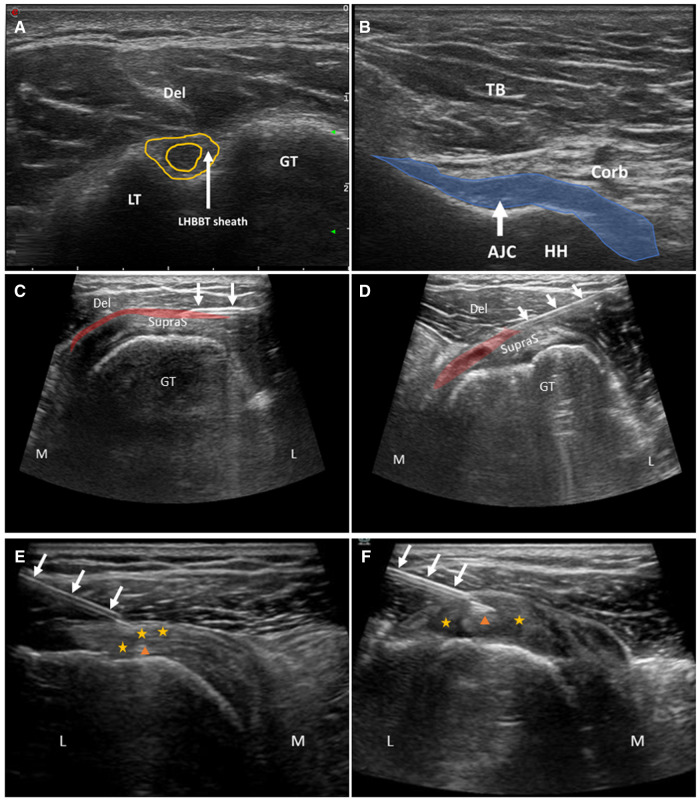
A,B are ultrasound images for diagnosis. C (before injection) and D (after injection) are US-guided injection into the subacromial Bursa. E (before injection) and F (after injection) are intrathecal injection into the LHBBT sheath. Del, deltoid muscle; TB, Triceps brachii; Corb, coracobrachialis; AJC, axillary joint capsule; HH, humeral head; GT, greater tubercle of humerus; LT, lesser tubercle of humerus; SupraS, supraspinatus tendon; L, lateral; M, medial; yellow star, rotator interval; orange triangle, the long head of the biceps brachii tendon (LHBBT); highlighted red area, subacromial Bursa. White arrows indicate the injection needle.
